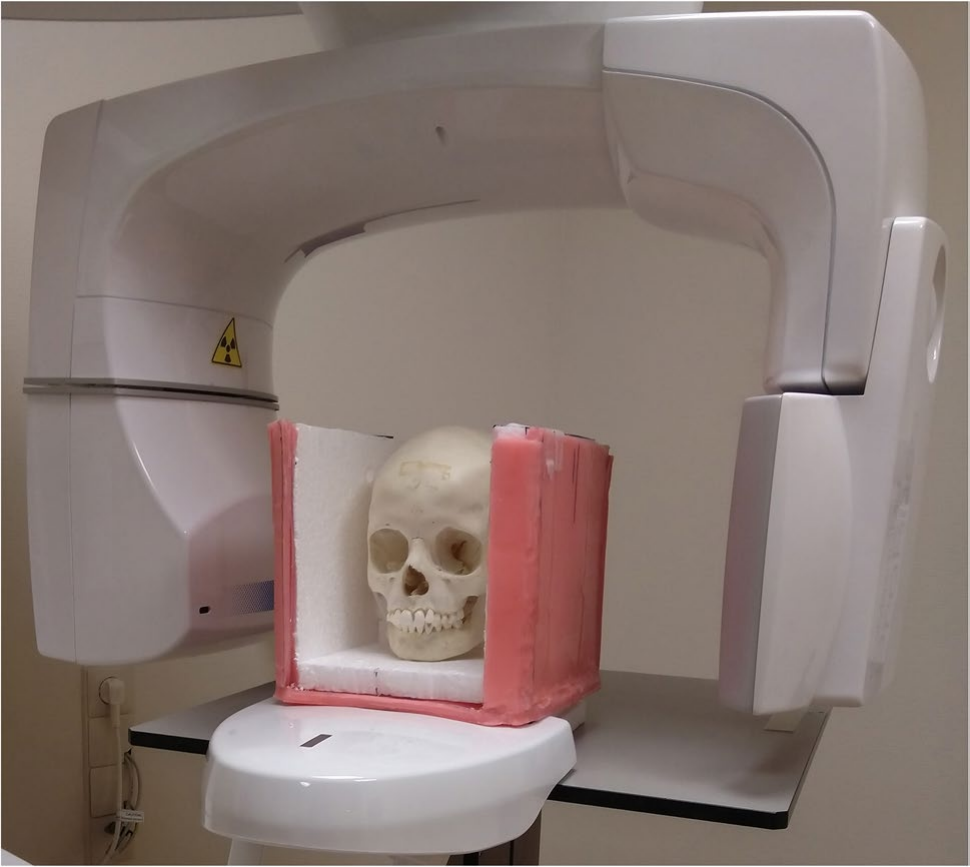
Dry skull in EPS box with 1-cm utility wax positioned in CBCT machine (front part removed for photo)
